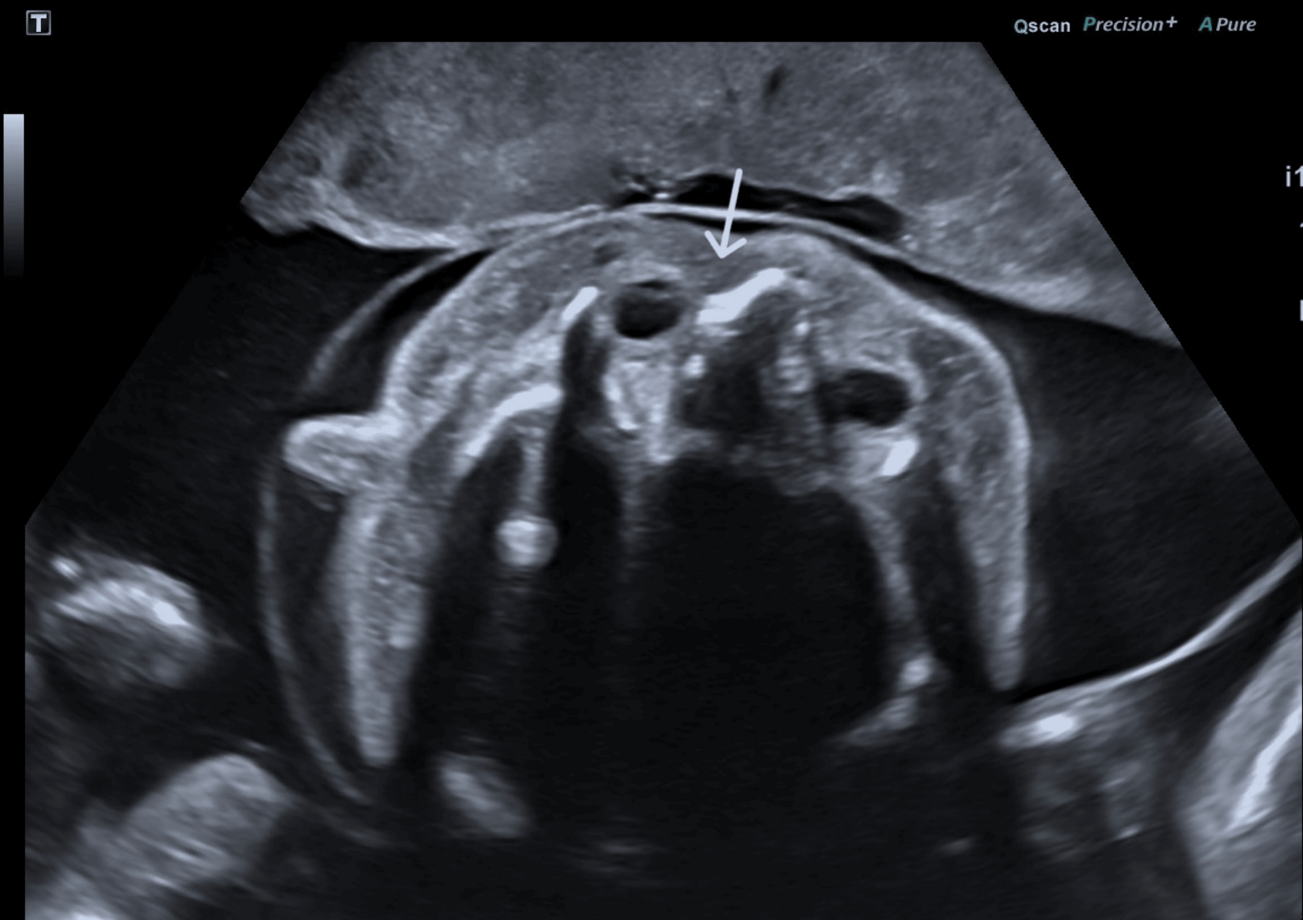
Transverse ultrasound view of the head demonstrating subcutaneous edema. White arrow: subcutaneous edema
